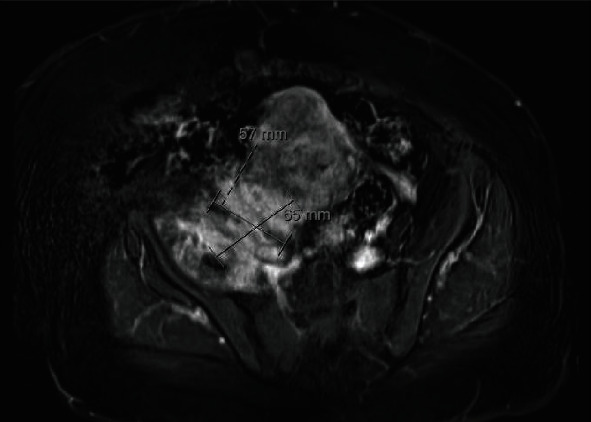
MRI of pelvis demonstrating a 6.5 × 5.7 cm ill-defined solid mass involving right iliopsoas muscle and extending to right uterus.
